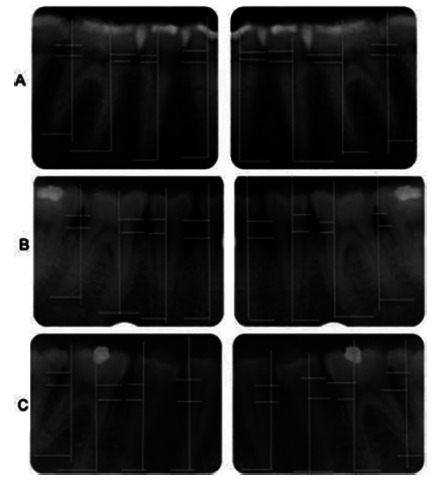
Right and left periapical radiographs in A eutrophic example
